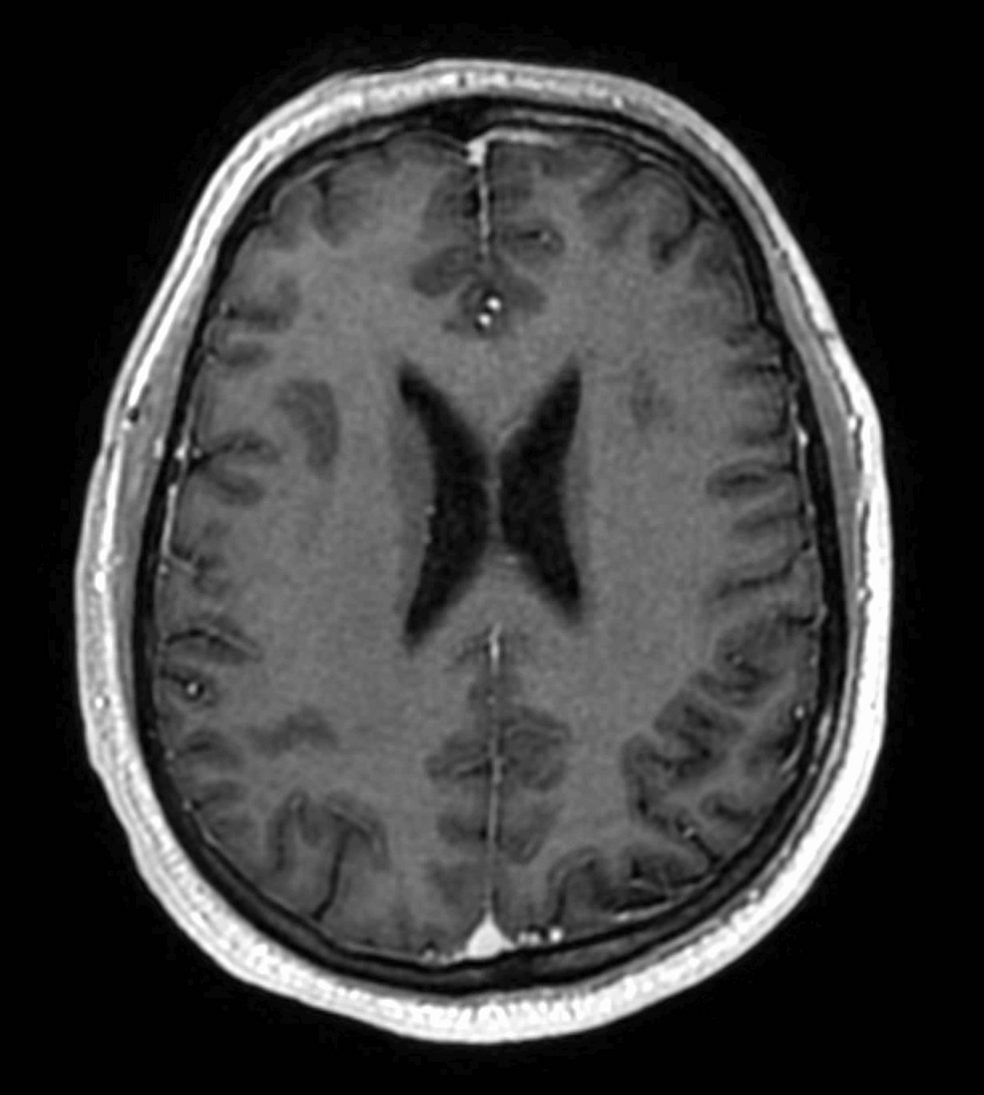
Axial head MRI T1 post-contrast at the level of the lateral ventricles showing no abnormal enhancement or signs of leptomeningeal disease
